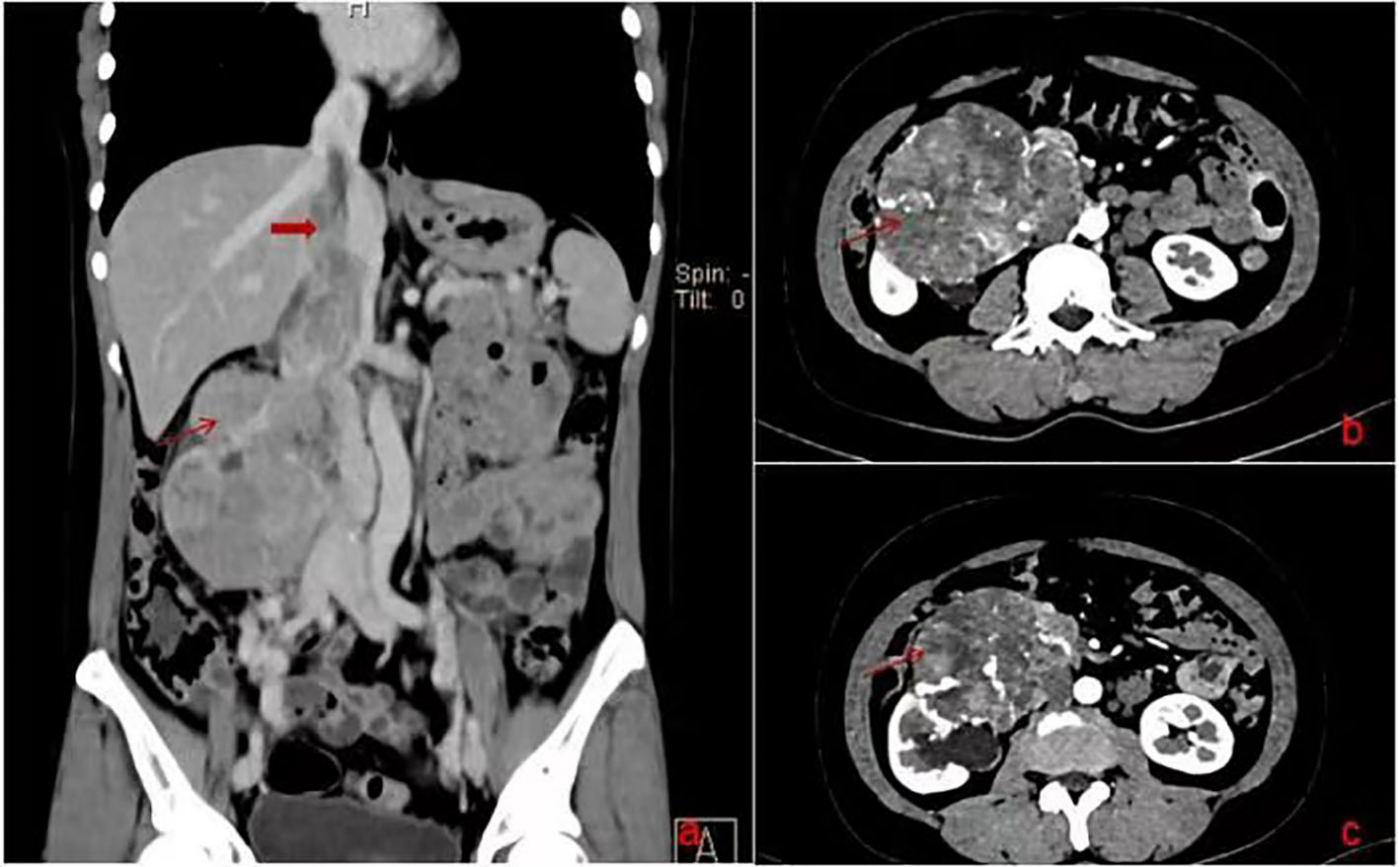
(A) is preoperative coronal CT. (B) and C is preoperative horizontal CT.
